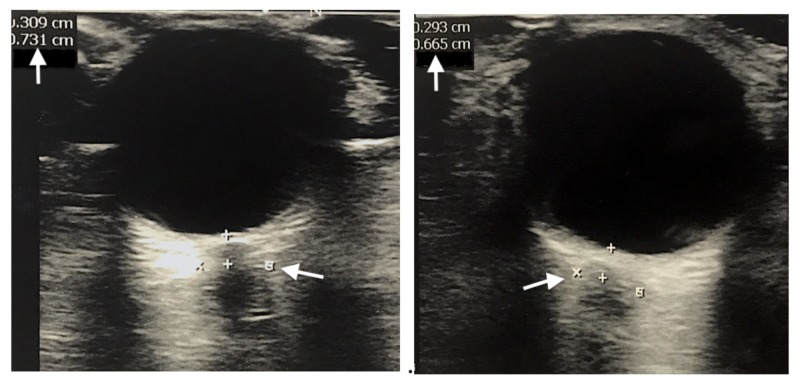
Bilateral Intra-ocular Ultrasound Optic nerve sheath diameter (ONSD) – the outer-to-outer diameter as measured 3 mm posterior to the globe (arrow): 7.31 mm in left eye (left image) and 6.65 mm in right eye (right image).
